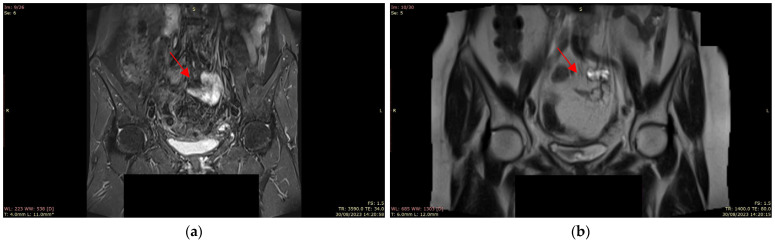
(a) Coronal post-contrast T1-weighted MRI showing a 20 mm enhancing submucosal lesion in the sigmoid colon (arrow), with well-defined margins and no adjacent invasion; (b) Coronal T2-weighted pelvic MRI showing a well-defined submucosal lesion in the anterior sigmoid colon wall (arrow), measuring approximately 17–22 mm, with intermediate-to-high signal intensity and no signs of adjacent invasion.
